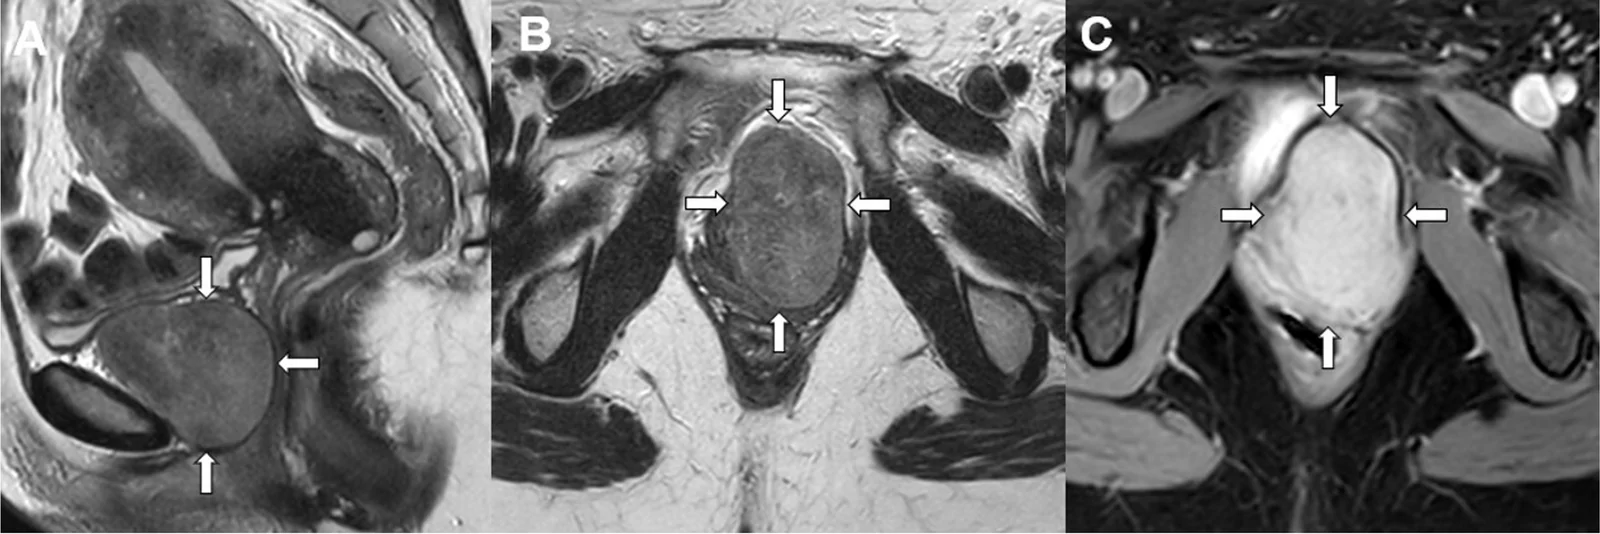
Periurethral leiomyoma in the space of Retzius. Sagittal and axial T2-weighted (A, B) and axial post-contrast T1-weighted (C) MR images demonstrate a T2-hypointense, homogeneously enhancing round mass in the space of Retzius (arrows)
